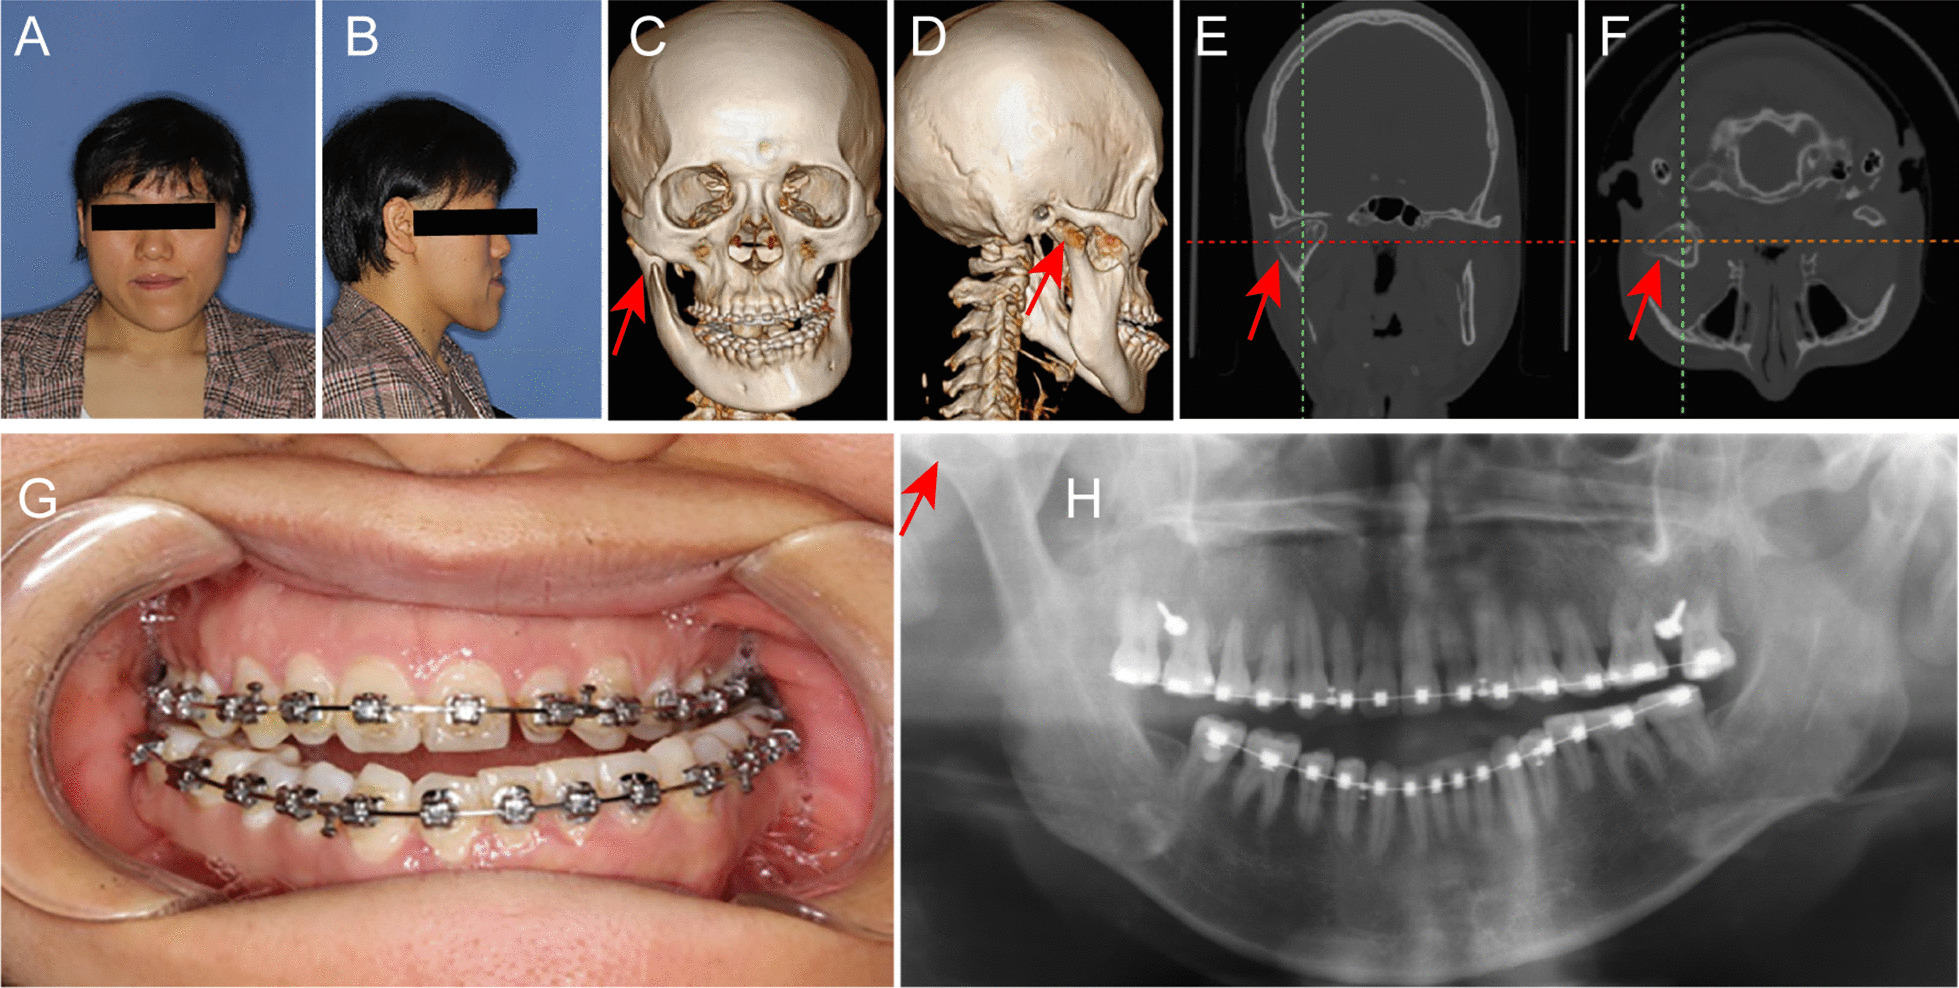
A woman with mandibular condylar osteochondroma. a Front profile; b lateral profile; c front three-dimensional CT image; d lateral three-dimensional CT image; e coronal CT scan; f horizontal CT scan; g intraoral view; h panoramic radiograph. Red arrow, condylar osteochondroma
